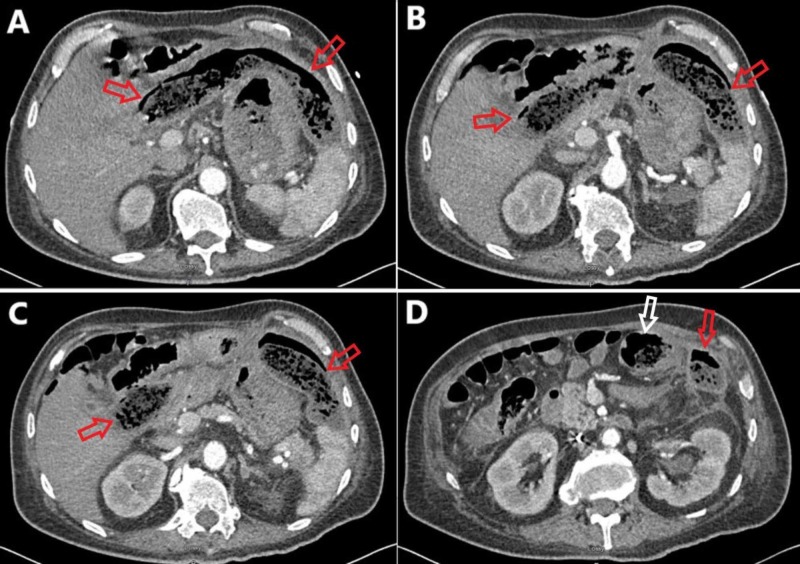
Contrasted computed tomography imaging of the abdomen and pelvis in the axial plane progressing from superior (A) to inferior (D). (A) A large, feculent phlegmon (red arrows) is apparent in the left upper quadrant anterior to the stomach and reaching the liver. (B) The phlegmon (red arrows) continues its inferior course in the abdomen. (C) The phlegmon spans into both the left and right upper abdominal quadrants (red arrows). (D) The phlegmon (red arrow) is in close proximity to the transverse colon (white arrow).
